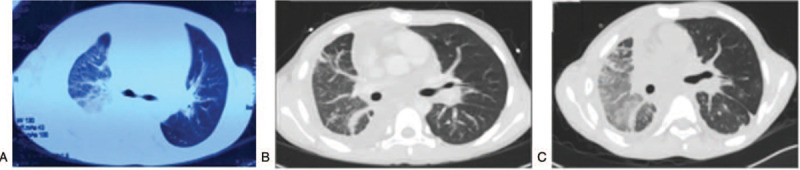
(A) Chest computed tomography (CT) in April 2012 showing a massive right pleural effusion, as well as patchy, dense patches. (B) Chest CT in February 2013 showing the collapse of the right thorax and left lower lung, and thickening of the lobular septum. (C) Chest CT in September 2017 showing the collapse of the right thorax; the texture of both lungs was disordered, and glassy density of the right lung was observed in all layers.
